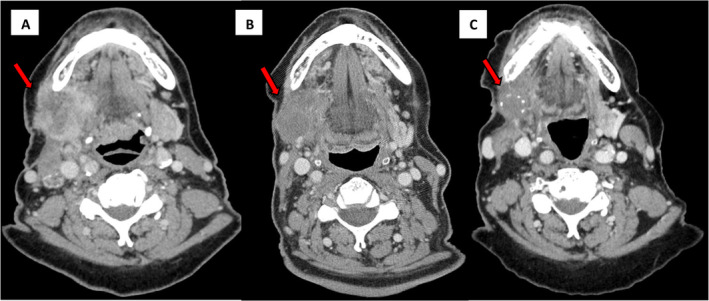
Head and neck computed tomography images. (A) CT scan revealed a solid mass of the right submandibular gland, infiltrating the right parotid gland and lower jaw, and bilateral cervical lymphadenopathy at baseline. (B) CT reexamination showed that both submandibular solid mass and cervical bilateral lymphadenopathy were significantly reduced after chemotherapy. (C) CT scan showed additional reduction of submandibular mass and cervical bilateral lymphadenopathy after 3 months of neratinib treatment.
